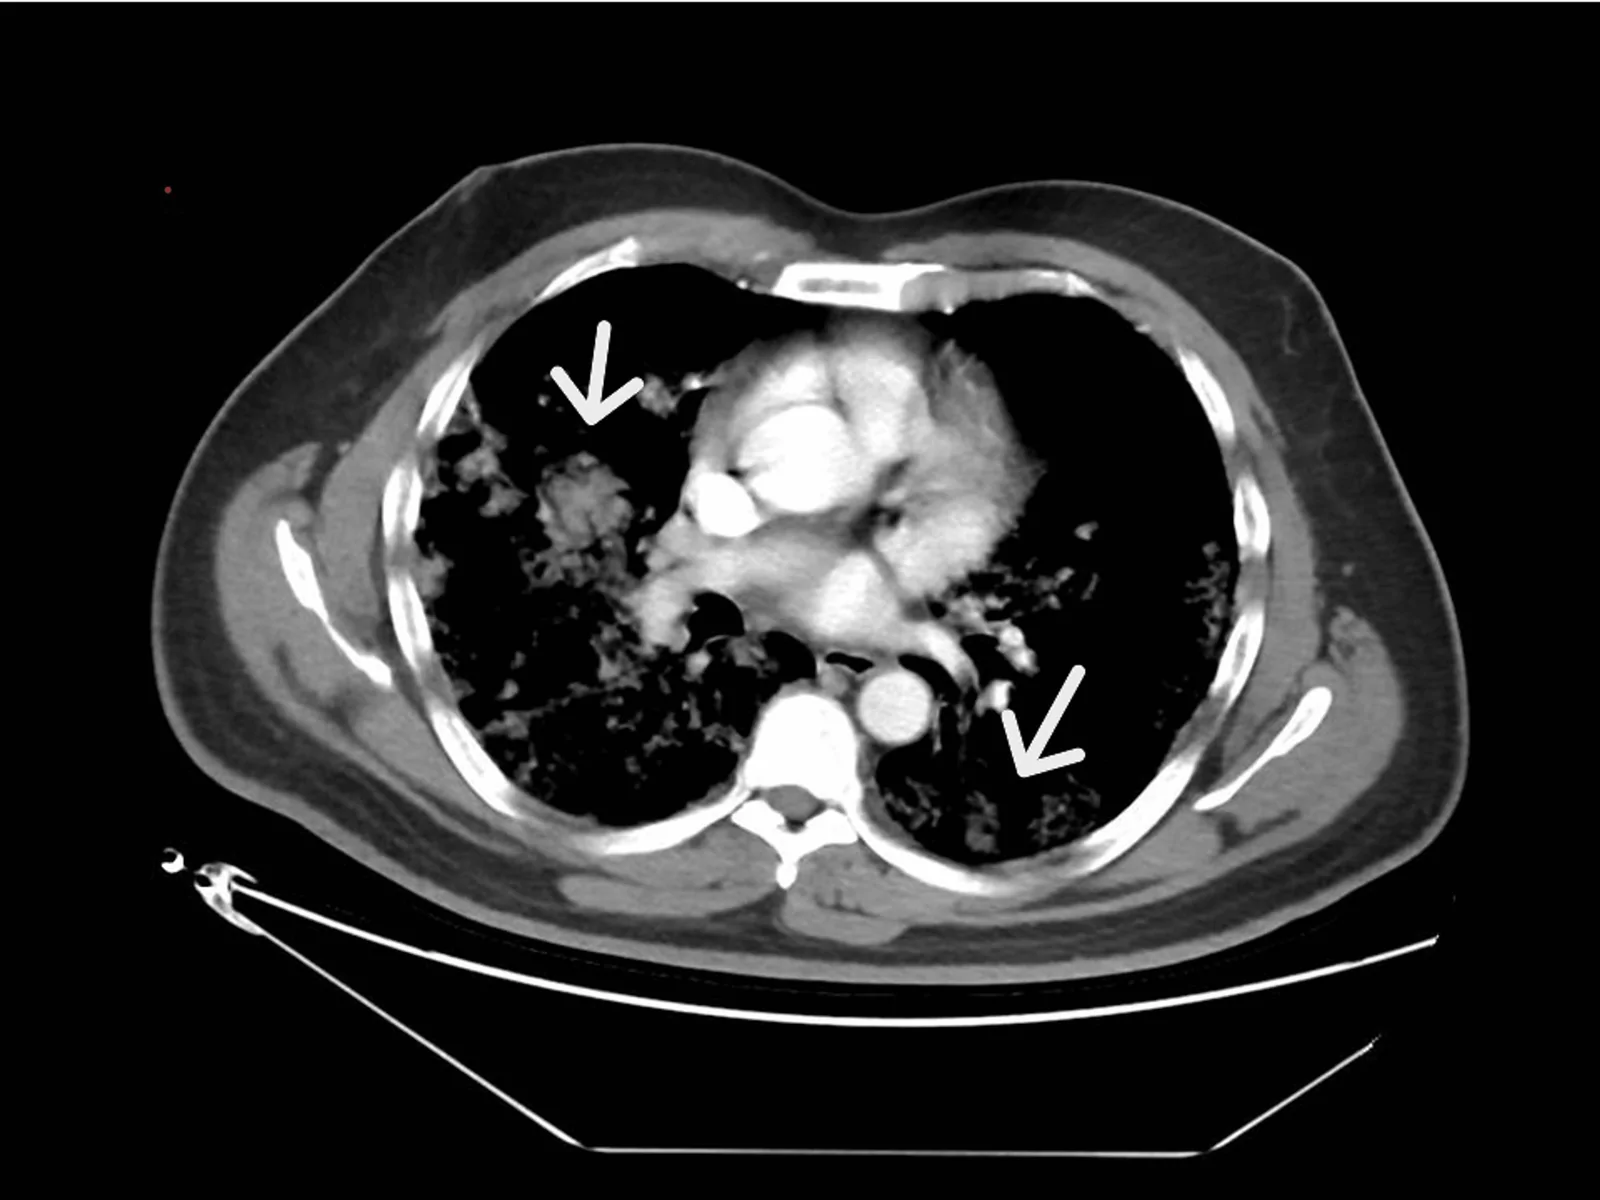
Axial Chest Computed Tomography of the Chest Axial chest computed tomography of the chest demonstrating bilateral multifocal air-space opacities, including patchy consolidative changes (arrows) and surrounding ground-glass attenuation, in keeping with diffuse alveolar haemorrhage in the clinical context of anti-glomerular basement membrane disease.
